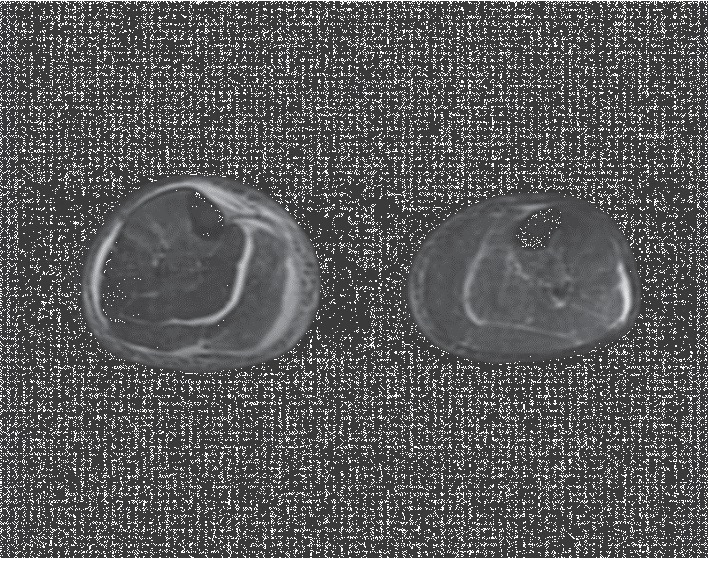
Horizontal section of magnetic resonance imaging (MRI) of lower extremities, with and without contrast, showing edema within the fascial compartments between the right gastrocnemius and right soleus muscles, as well as in the superficial fascia. A crescent-shaped subfascial fluid collection was also noted over the medial head of the right gastrocnemius muscle, indicative of fasciitis.
